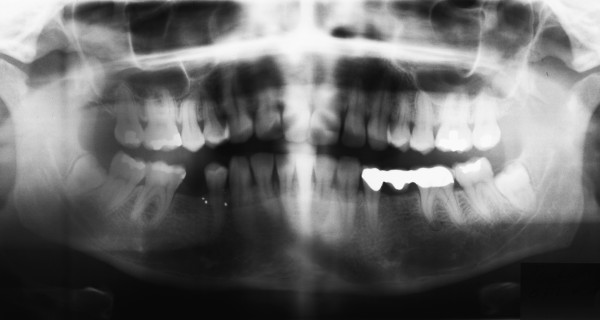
Pre-operative panoramic radiograph of the right mandibular third molar in place with no cyst-like radiolucency.
